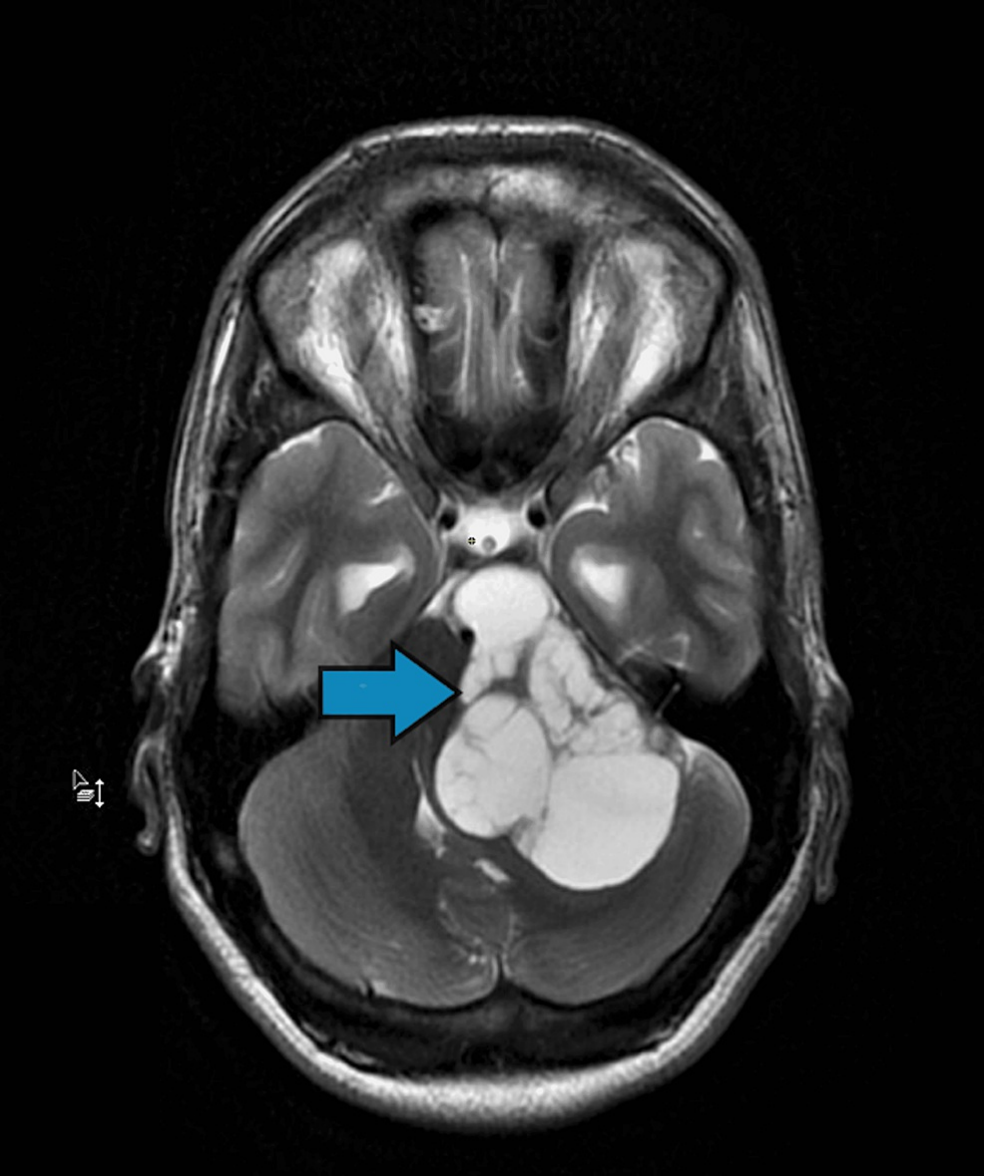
T2-weighted MRI findings showed a large lobulated cystic mass in the left CP cistern (size: 6.4 cm AP diameter and 3.97 TR) (blue arrow). Compression of the brainstem, vermis and ipsilateral cerebellar hemisphere was found. MRI: magnetic resonance imaging; CP: cerebellopontine; AP anteroposterior; TR: transverse; IAC: internal auditory canal
